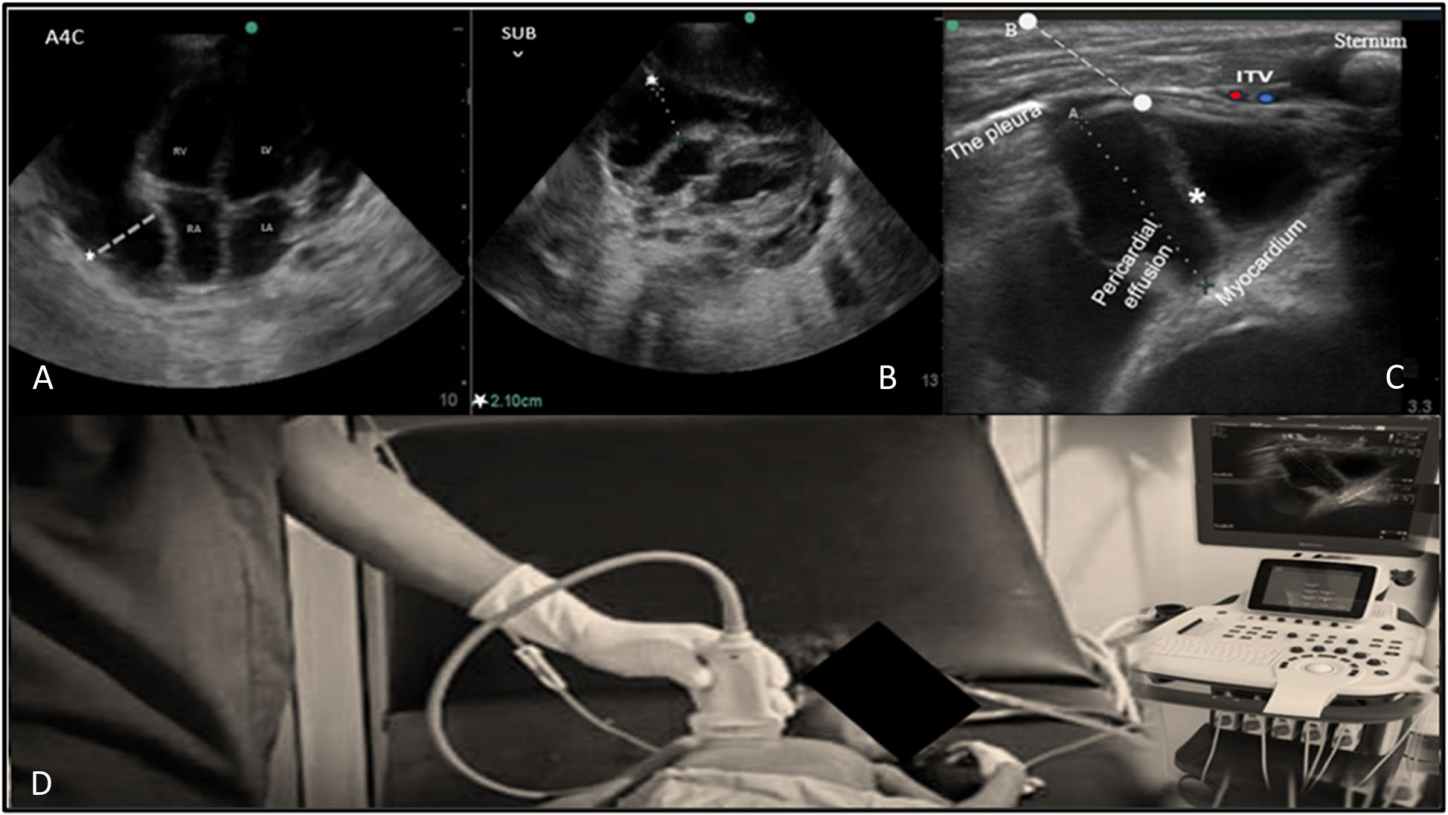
A, B Pre-procedural echocardiography views (apical 4-chamber and subxiphoid) revealing a large pericardial effusion concentrated maximally around the right atrium (dashed line and star). C Right parasternal ultrasonographic view displaying the pericardial effusion with fibrin strands, the surrounding vital structures (pleura, internal thoracic vessels (ITVs), and myocardium), the diameter of cardiac effusion *A*, and the path of needle insertion *B* (the distance from the skin to the pericardial space). D Image demonstrating the position of operator, ultrasound machine, and placement of high-frequency ultrasound probe in the right parasternal area
